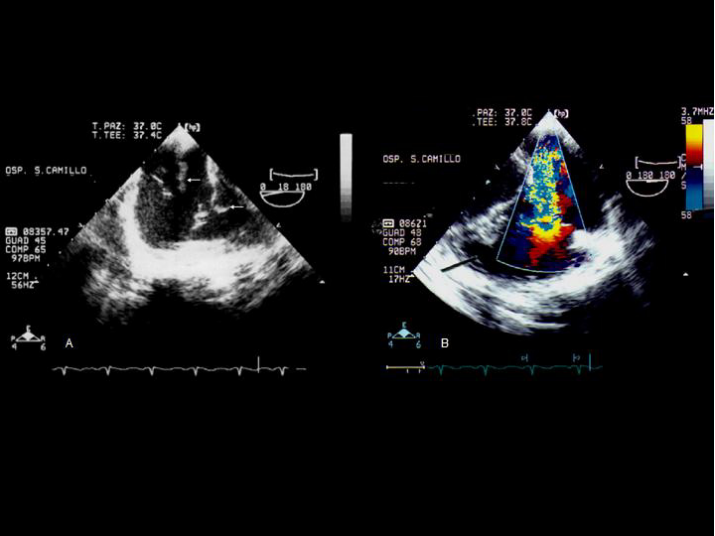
A Transesophageal echocardiographic view showing two peduncolated vegetations attached to the eustachian valve and another vegetation on tricuspid valve. B, Color flow doppler echocardiogram which confirms a moderate tricuspid regurgitation with flow toward the eustachian valve.
